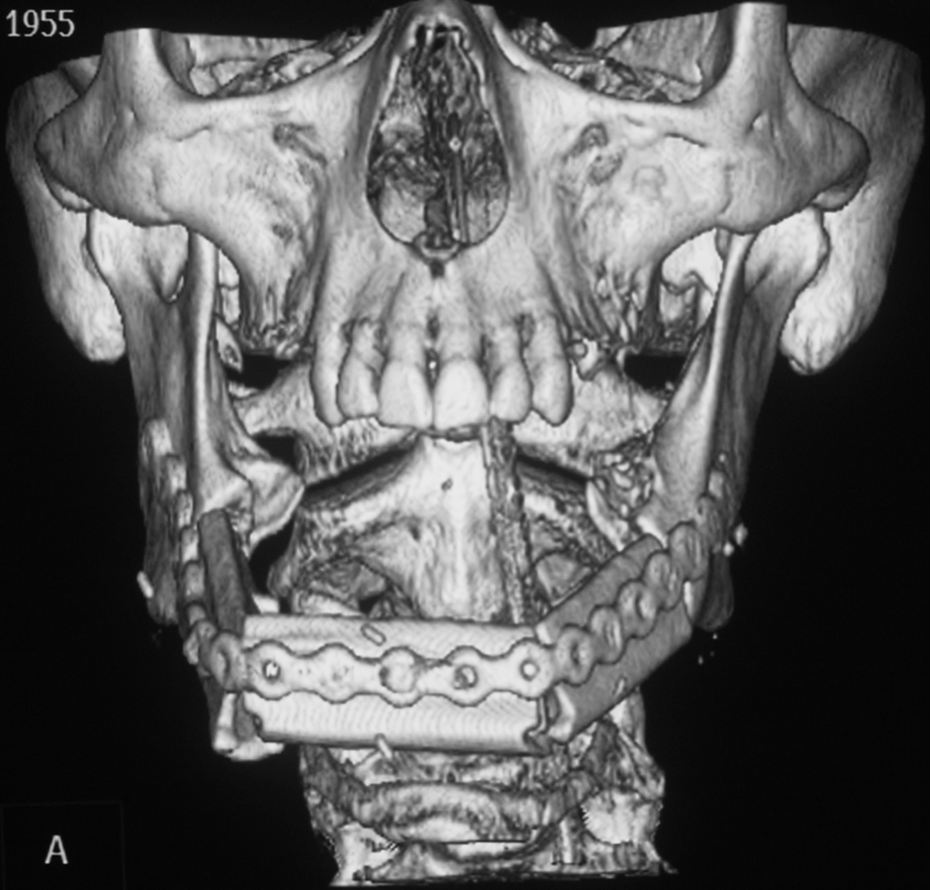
Fibula osteocutaneous flap for reconstruction of the mandible. CT reconstruction of the mandible 4 month after transplantation
